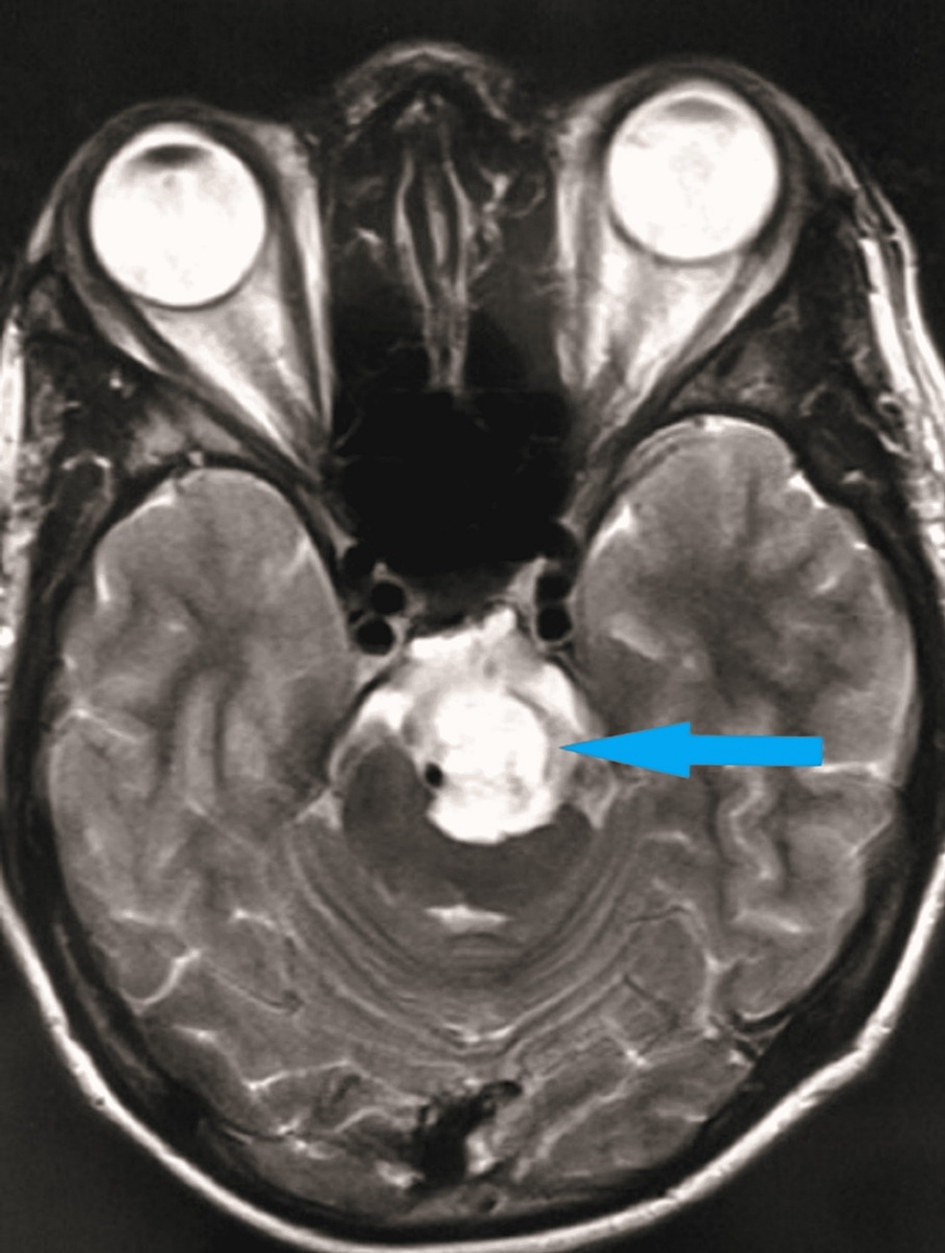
T2 axial MRI scan of the brain shows a hyperintense lesion in the pre-pontine cistern indenting the brainstem. MRI: magnetic resonance imaging
